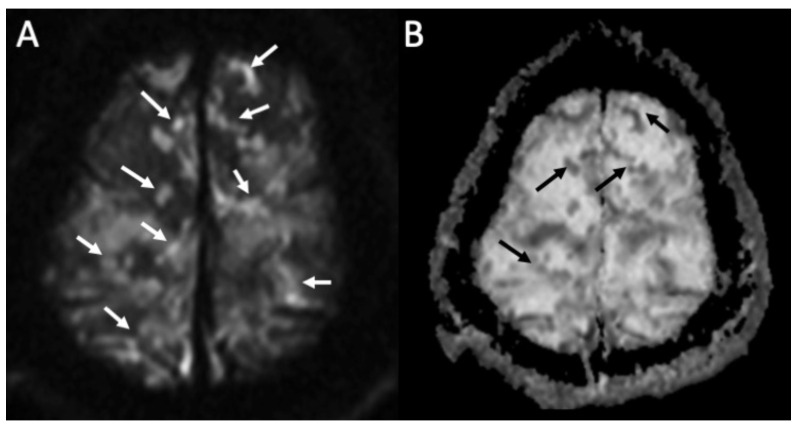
Axial DWI (A), ADC (B) shows multiple foci of restricted diffusion in the subarachnoid spaces at convexity bilaterally (arrows in A,B) in a patient with newly diagnosed Streptococcus Pneumoniae meningitis.
